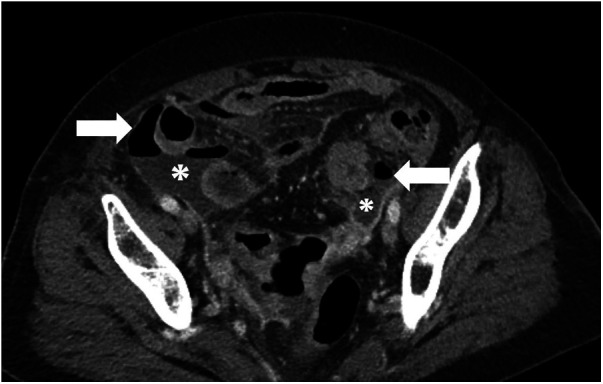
Abdominal CT findings suggestive of a pelvic abscess secondary to oophoritis. The image reveals pneumoperitoneum (arrow) and a large pelvic fluid collection, consistent with an abscess (asterisk).
